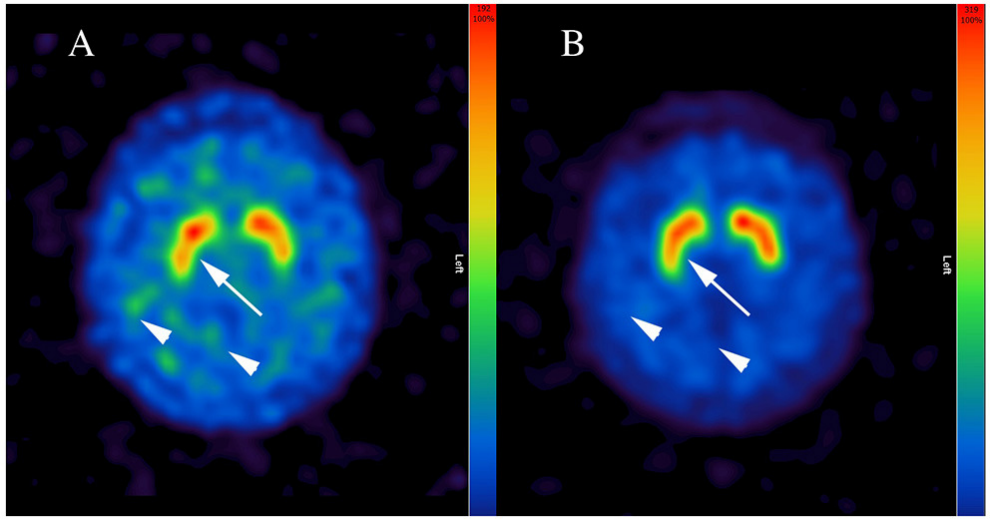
Representative [^123^I]FP-CIT-SPECT images of the patient. Note inhomogeneous and reduced dopamine transporter binding of the radiopharmaceutical in putamen bilaterally (arrow in A) and low contrast between striatal and cortical binding during short-term bupropion therapy (arrowheads in A), which normalized after a wash-out phase of four half-life periods (arrow and arrowheads in B). Bupropion reduced the binding of [^123^I]FP-CIT up to 45% (i.e., in right putamen).
